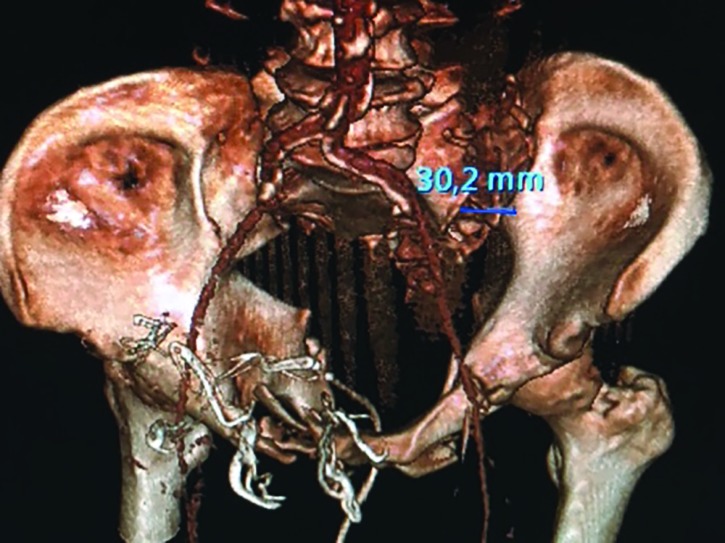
3D-CT image of a patient with residual displacement > 30 mm of the left sacroiliac-joint
